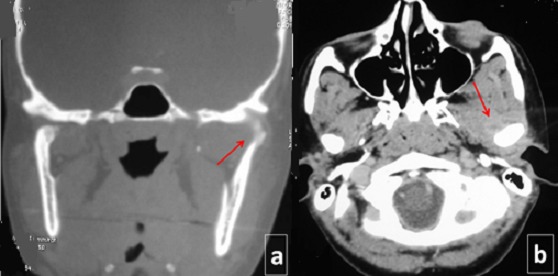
A) CT scan, 1 year later, showing osteolysis of the left condyle; B) without reactivation in the other side and abcesses in soft tissue
